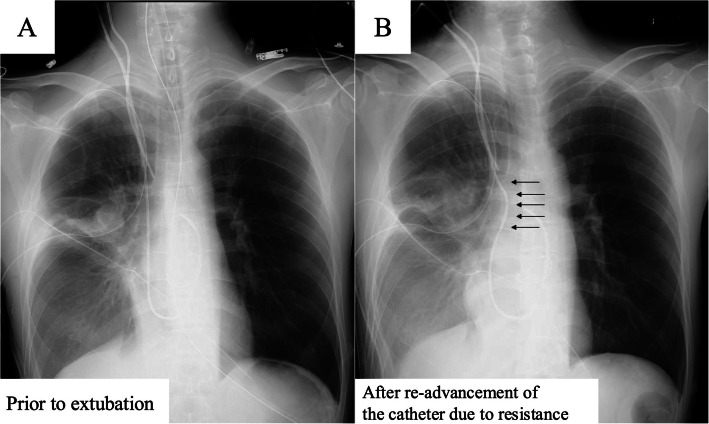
Pulmonary artery catheter positioning on chest radiographs. A Prior to extubation. B After re-advancement of the Pulmonary artery catheter due to resistance. Black arrow indicates abnormal curvature of the Pulmonary artery catheter near the superior vena cava
